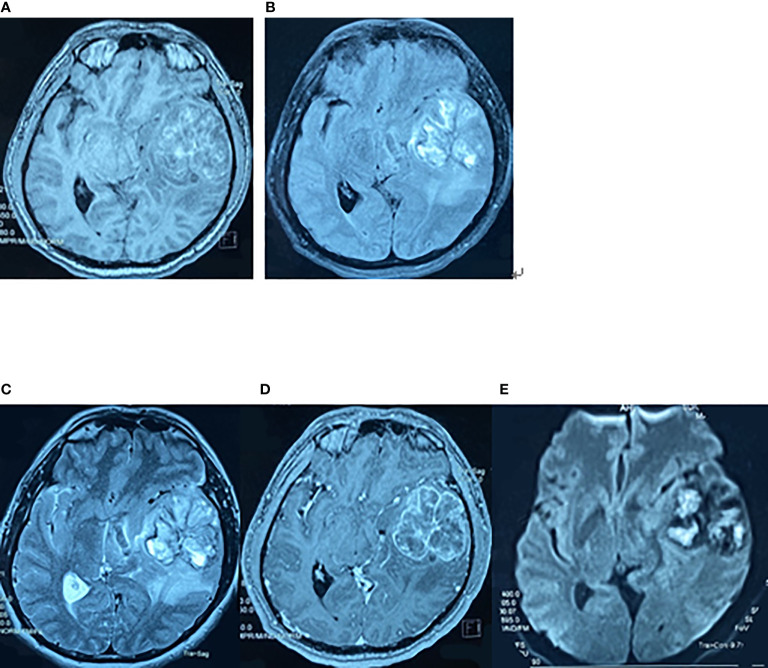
Preoperative brain MRI findings on May 7, 2017. The axial planes of preoperative MRI of the brain showing a 6-cm heterogeneous mass in the left superior temporal lobe. (A) T1-weighted MRI. (B) T2-weighted fluid-attenuated inversion recovery (FLAIR) MRI. (C) T2-weighted MRI. (D) Enhanced T1-weighted MRI. (E) Diffusion-weighted MRI.
